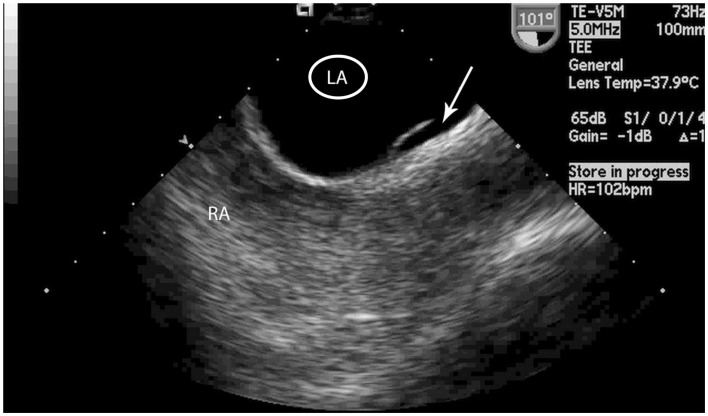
Transesophageal echocardiogram image of the left atrial septal pouch. Transeophageal echocardiogram image of the left atrial septal pouch (arrow). LA, left atrium, RA, right atrium.
